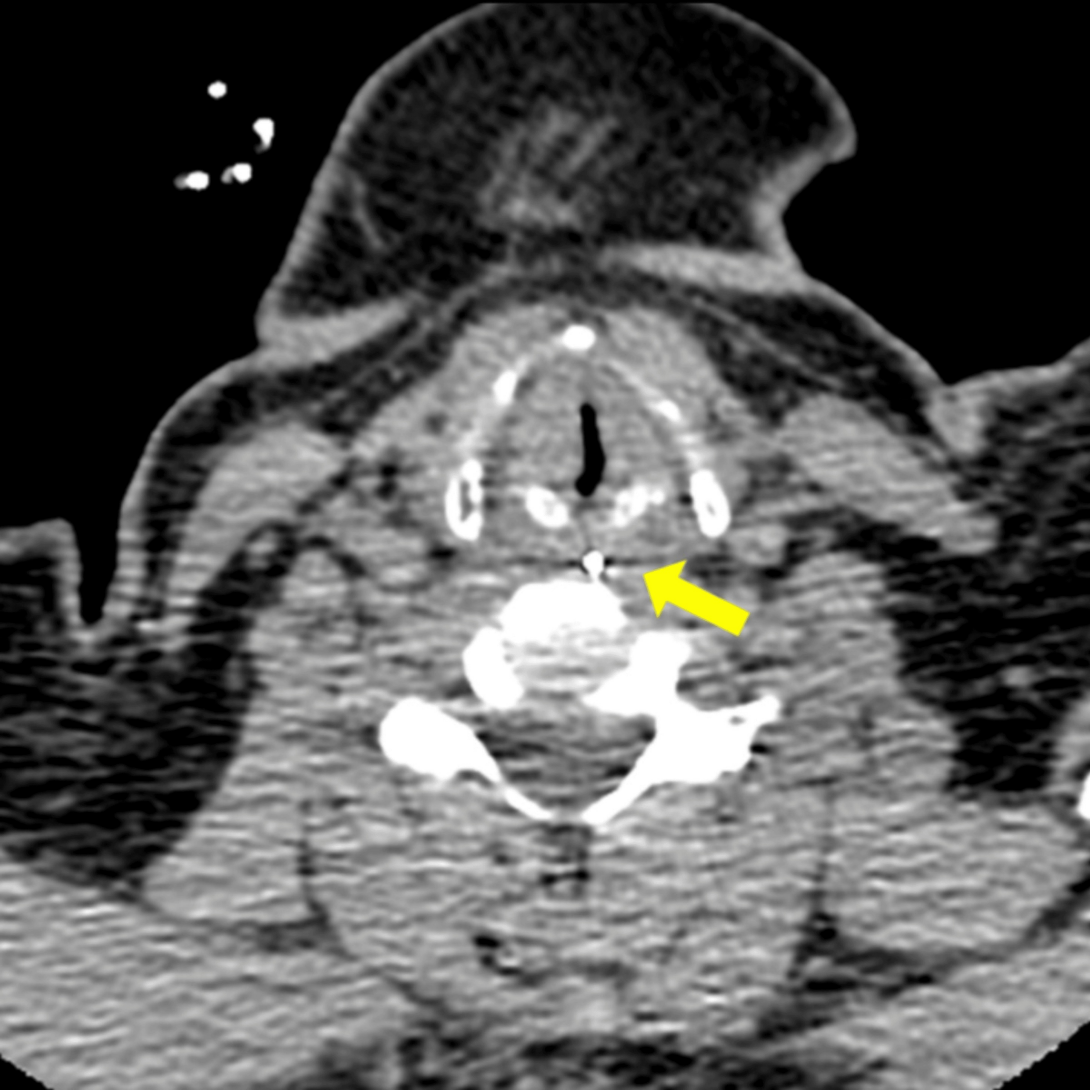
CT showing the nasogastric tube passing almost midway through the bilateral piriform sinuses (yellow arrow)
